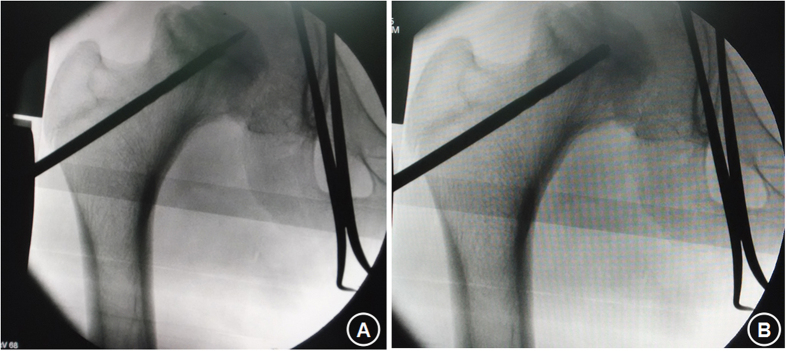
Intraoperative image showing core decompression treatment of ONFH. The decompression was done with a percutaneous approach using a 1.0-mm diameter Kirschner needle under X-ray guidance. Fluoroscopy-guided positioning of the pilot Kirschner wire into the necrotic lesion was performed in the AP and lateral plain view radiographs. Usually multiple small-diameter drill holes were made. Guided by the Kirschner wire, the hollow trephine was gently passed through the sclerotic zone of the necrotic lesion under fluoroscopic control in the anteroposterior (A) and lateral (B) plain view radiographs. The trephine should be 2–3 mm away from the cartilage. Debridement of the necrotic lesion was done through the drill hole.
